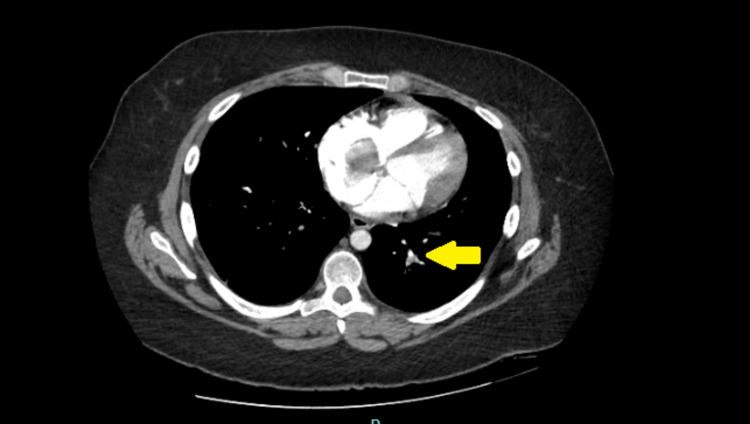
CT of the chest with contrast showing a small left lower lobe pulmonary embolism (yellow arrow). CT: computed tomography
